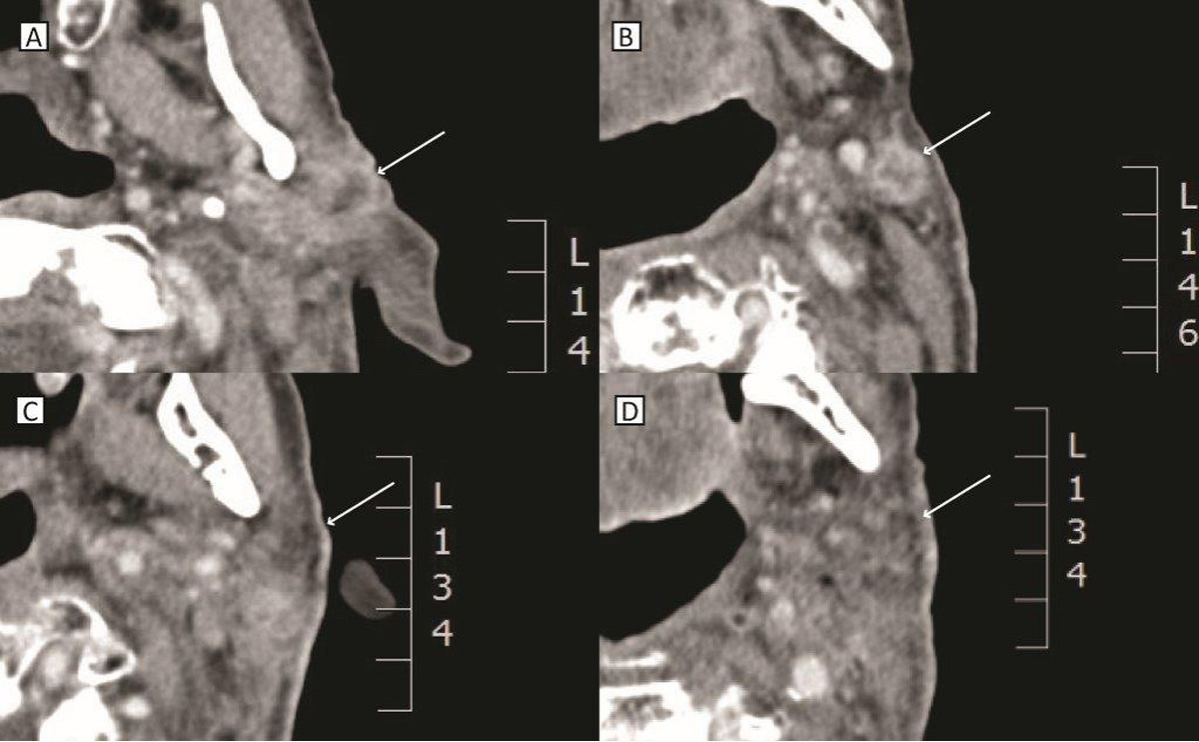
CT images before (A, B) and after (C, D) electrochemotherapy. PR of the metastasis No. 1 in the left parotid gland (A, C). CR of the metastasis No. 2 behind the left mandibular angle (B, D). Locations of the metastases are marked with arrows.
